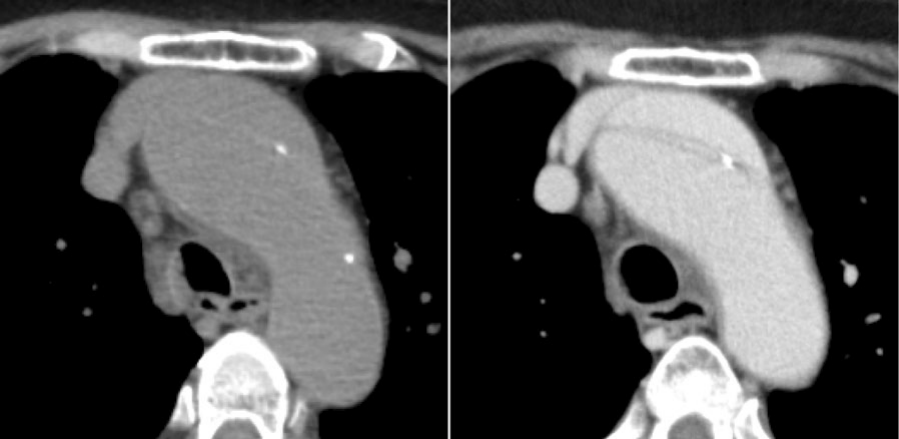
The axial view of the plain (left) and contrasted (right) CT scan of case 2. Note the presence of a displaced and slightly calcified intimal flap in the plain CT scan, which was confirmed with contrast
